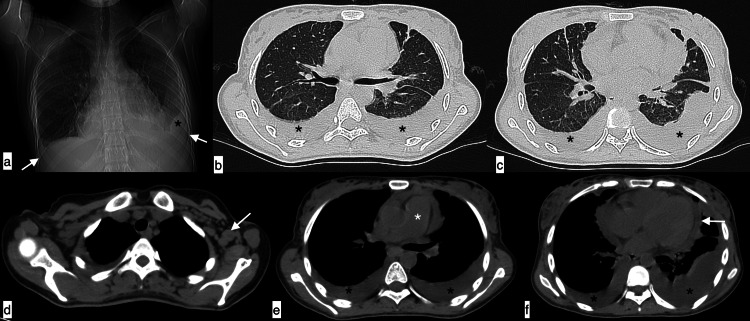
Serositis A 29-year-old female patient with SLE presented with chest pain, fever, and cough of seven-day duration. The topogram (a) revealed blunting of bilateral costophrenic angles (white arrows) with suggested loculated pleural effusion on the left side (black asterisk). Axial chest HRCT images (b-f) confirmed bilateral moderate pleural effusions (black asterisk), pericardial effusion (f, white arrows), cardiomegaly, enlarged pulmonary artery (e, white asterisk), and axillary lymphadenopathy (d, white arrow). Features were suggestive of serositis. SLE: systemic lupus erythematosus; HRCT: high-resolution CT
